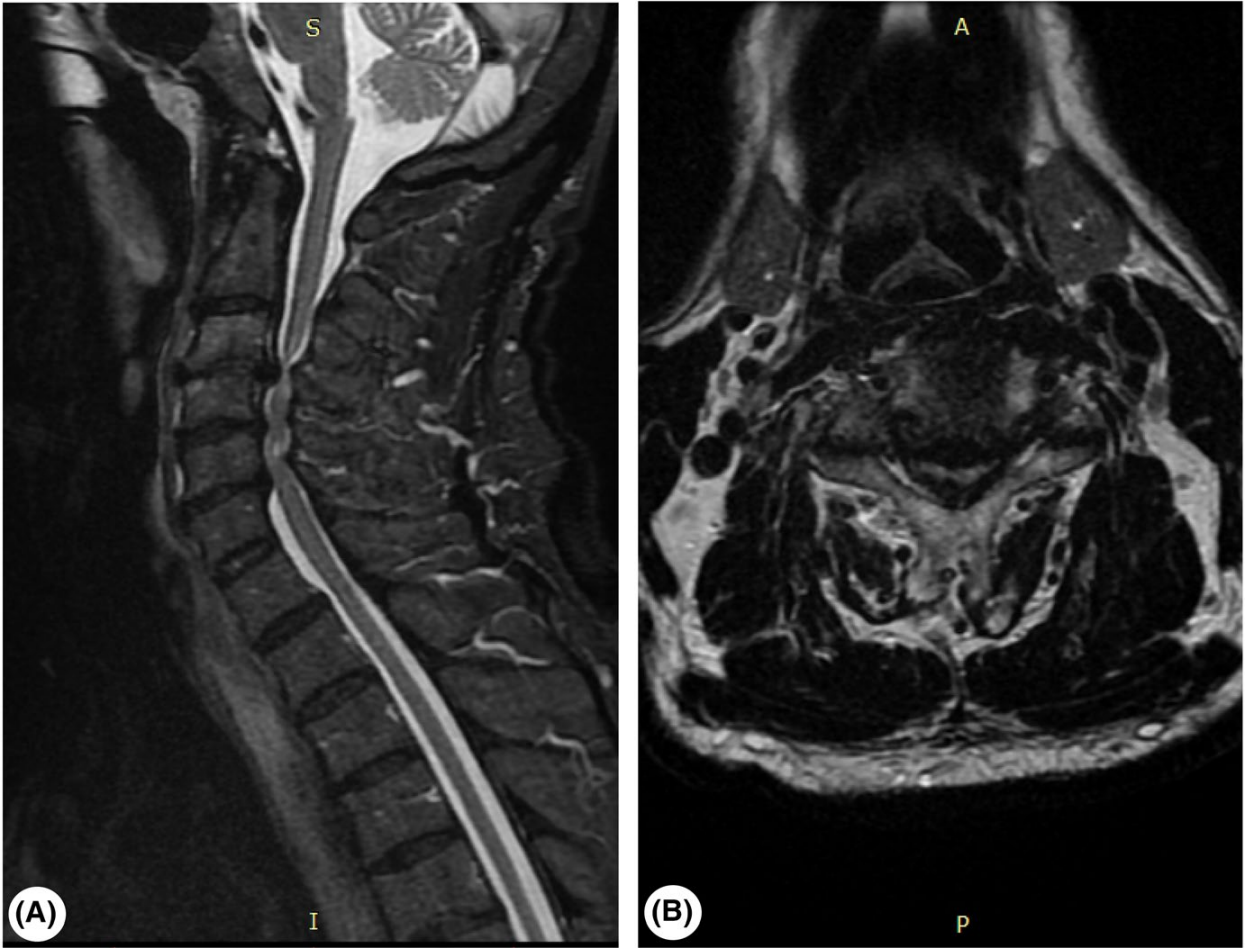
Preoperative cervical nonenhanced MRI aspect. A, Sagittal STIR sequence, demonstrating significant compressive spinal cord suffering from multiple disk herniations and severe stenosis of the spinal canal. B, Axial T2‐weighted frFSE sequence, revealing posterior herniation of the cervical disk, compressing both the spinal cord and spinal nerve roots
